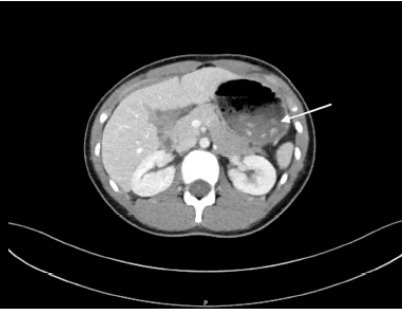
Axial computed tomography with arrow demonstrating radiopaque foreign bodies within the stomach.
